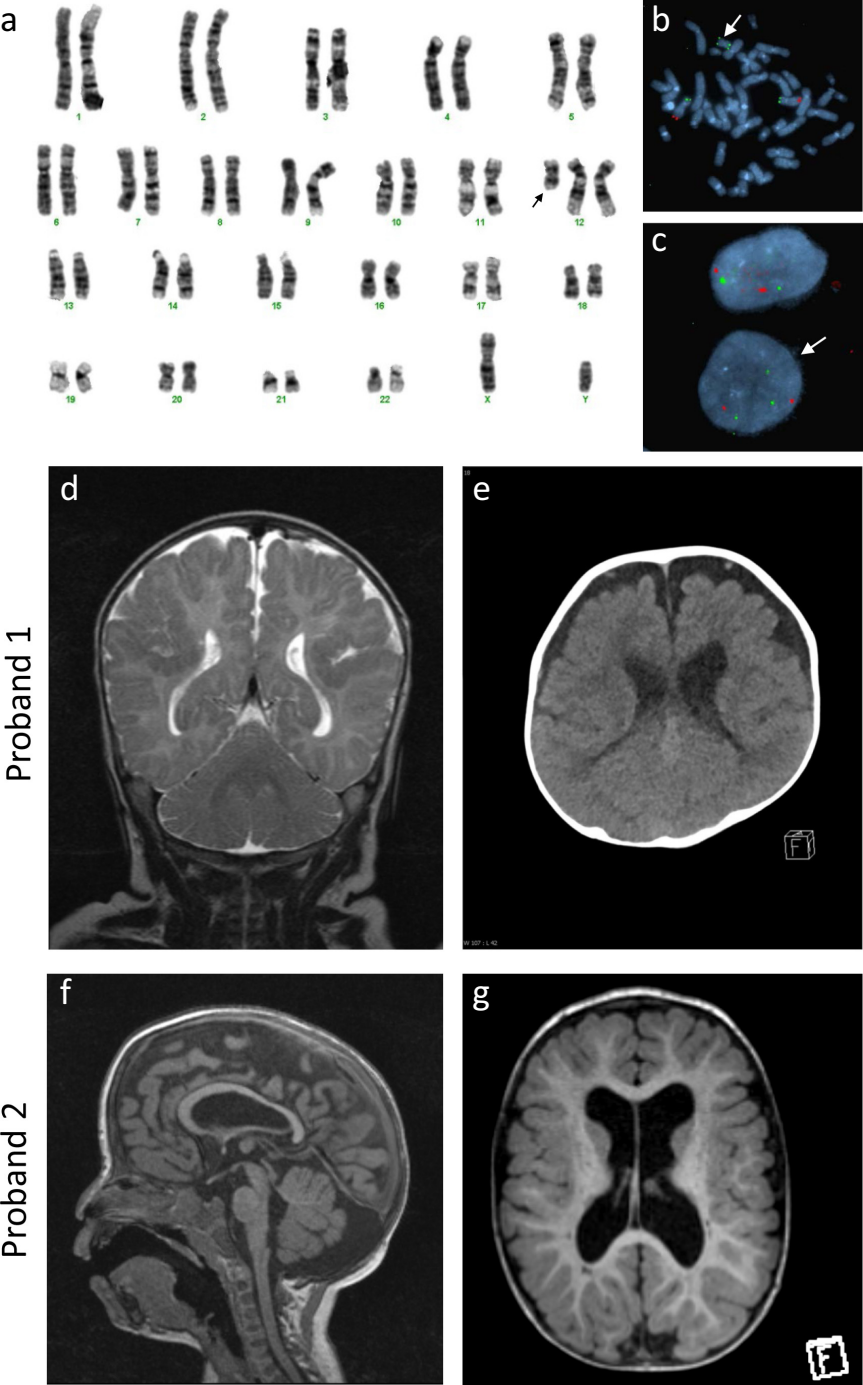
Pallister‐Killian syndrome with structural brain abnormalities. (a) G‐banded chromosome analysis from cultured amniocytes from Proband 1 showed a male karyotype with a supernumerary marker chromosome (shown by the arrow). (b) Metaphase FISH analysis of cultured amniocytes from Proband 1 using subtelomeric probes for chromosome 12p13.33 (RP11‐283I3) (green signal) and 12q24.33 (RP11‐46H11) (red signal) showed the supernumerary marker chromosome with two green signals and indicated isochromosome 12p. (c) Interphase FISH analysis of skin fibroblasts from Proband 2 indicated mosaic trisomy 12p with the presence of three green signals for 12p13.33 (RP11‐283I3) and two red signals for 12q24.33 (RP11‐46H11) in approximately 14% of cells (shown by the arrow). (d–e) MRI and CT scans, respectively, reveal bilateral polymicrogyria in Proband 1. (f–g) MRI representation of thin corpus callosum and a prominent cisterna magna as well as enlarged lateral ventricles in Proband 2
